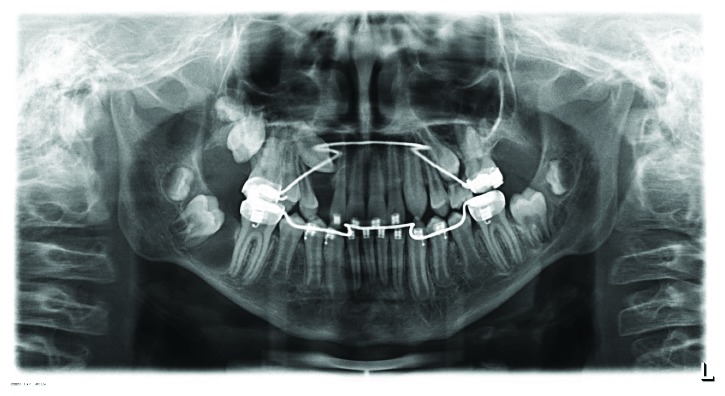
Panoramic radiograph (August 2014). Evaluation of the region of tooth 13. No manifest change and therefore no indication for further therapy. Radiolucency around the crown of the retained tooth 47, with indication for cystectomy.
